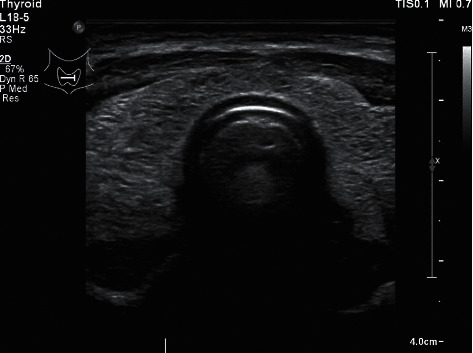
Thyroid ultrasonography of the patient demonstrating thyroid enlargement and inhomogeneity. The size of the left lobe was 1.5 × 1.6 × 2.8 cm, and the size of the right lobe was 1.7 × 1.5 × 3.5 cm. Anterior from both thyroid lobes was fluid accumulation.
